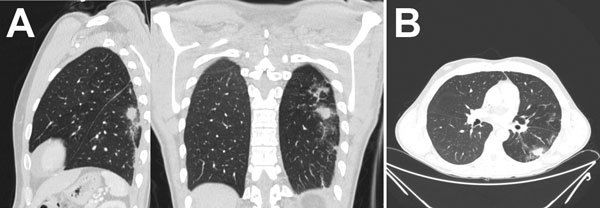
Computed tomography of the lungs in patient with invasive fungal disease caused by *Inonotus* spp., Madrid, Spain. Images show pulmonary nodule with halo sign in the left superior lobe, with peripheral distribution.
